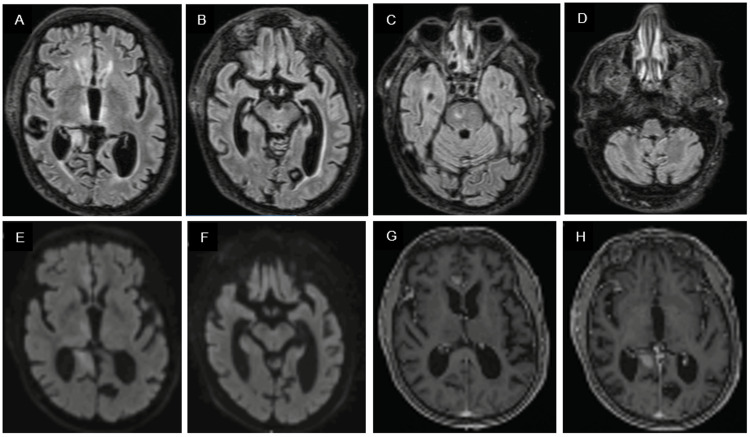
Brain MRI (two weeks later) revealing T2/FLAIR hyperintense lesions involving the thalami, midbrain, bilateral cingulate gyri, frontobasal and right anterotemporal basal regions, as well as the genu of the corpus callosum (A-D), with diffusion restriction on DWI (E and F) and contrast enhancement on post-contrast T1-weighted images (G and H). FLAIR: Fluid-attenuated inversion recovery
